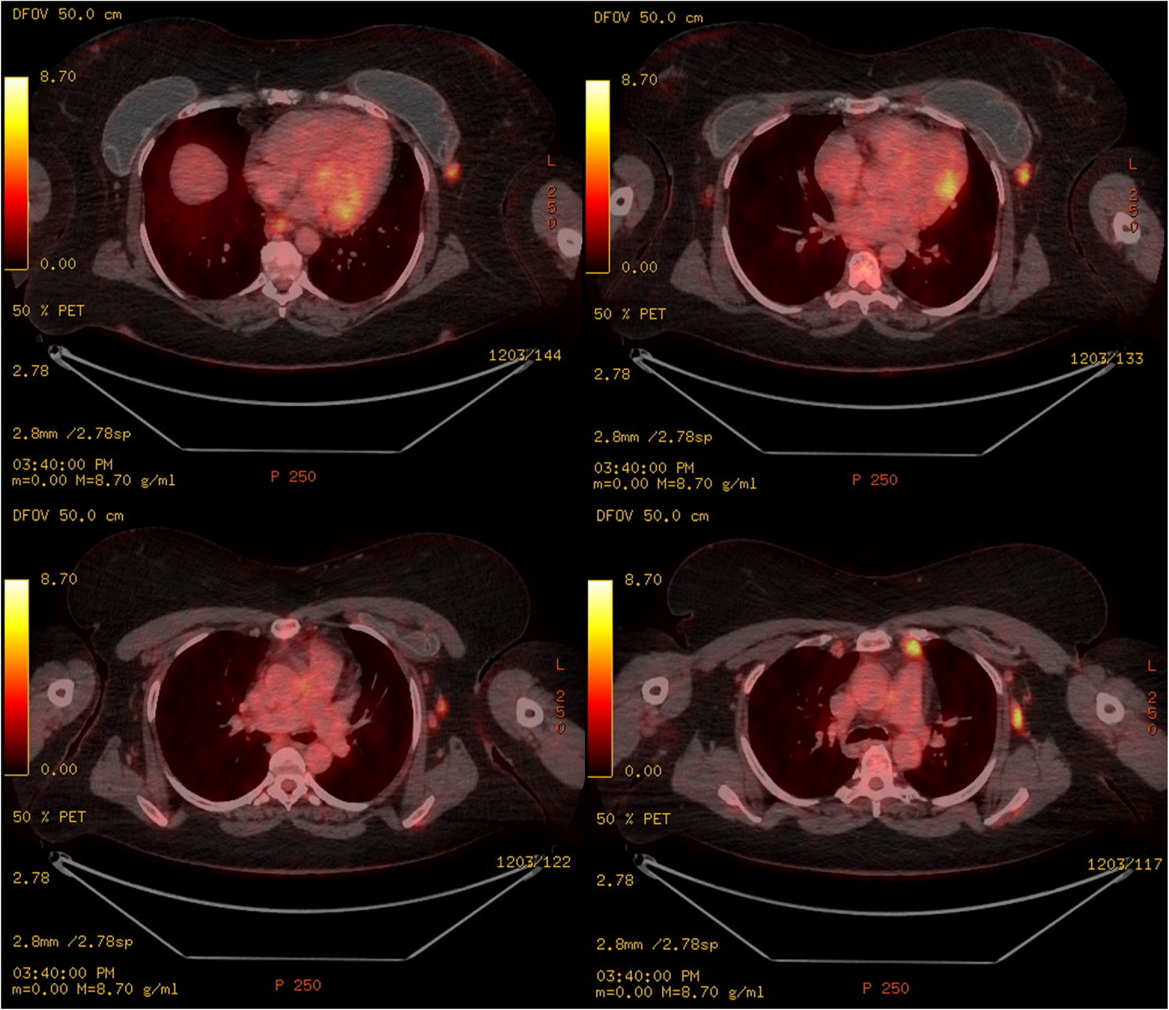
PET-CT imaging. PET-CT displaying hypermetabolic, enlarged lymph nodes, predominantly at the left side. Heterogenic content of the subpectoral breast prosthesis, suggestive of rupture
